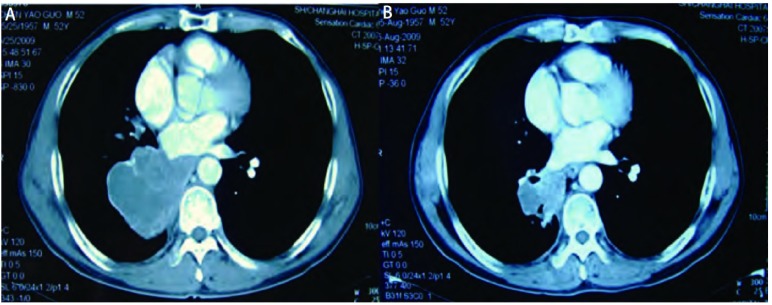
患者治疗前后胸部影像学改变。A：治疗前右下肺肿瘤最大直径为7 cm；B：两次化疗后肿瘤最大直径为3 cm。 The appearance of the chest computed tomogram. A: The diameter of tumor in right low lung was 7 cm before chemotherapy; B: The diameter of tumor in right low lung was 3 cm after two times chemotherapy.
